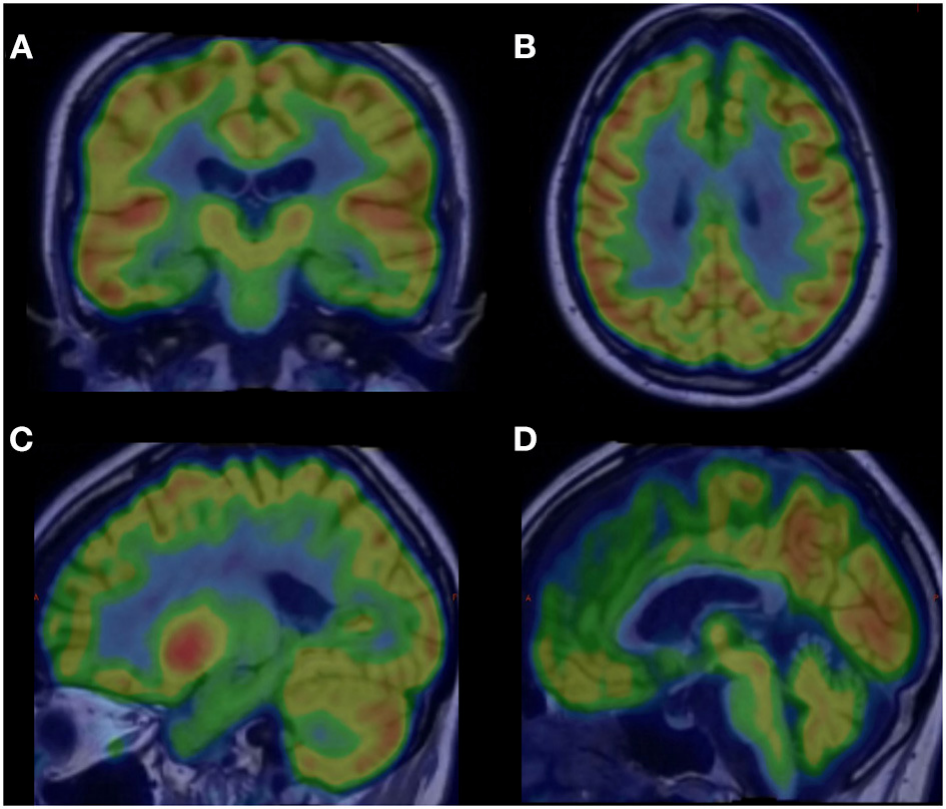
18F-FDG PET/CT slices exhibiting hypometabolism areas (green colored) in FTD: (A) hippocampus and mesial temporal lobes bilaterally (coronal slice); (B) medial frontal cortex/parafalcine region (axial slice); (C) precuneus and posterior cingulate cortex and (D) cerebellar vermis (sagittal slices).
